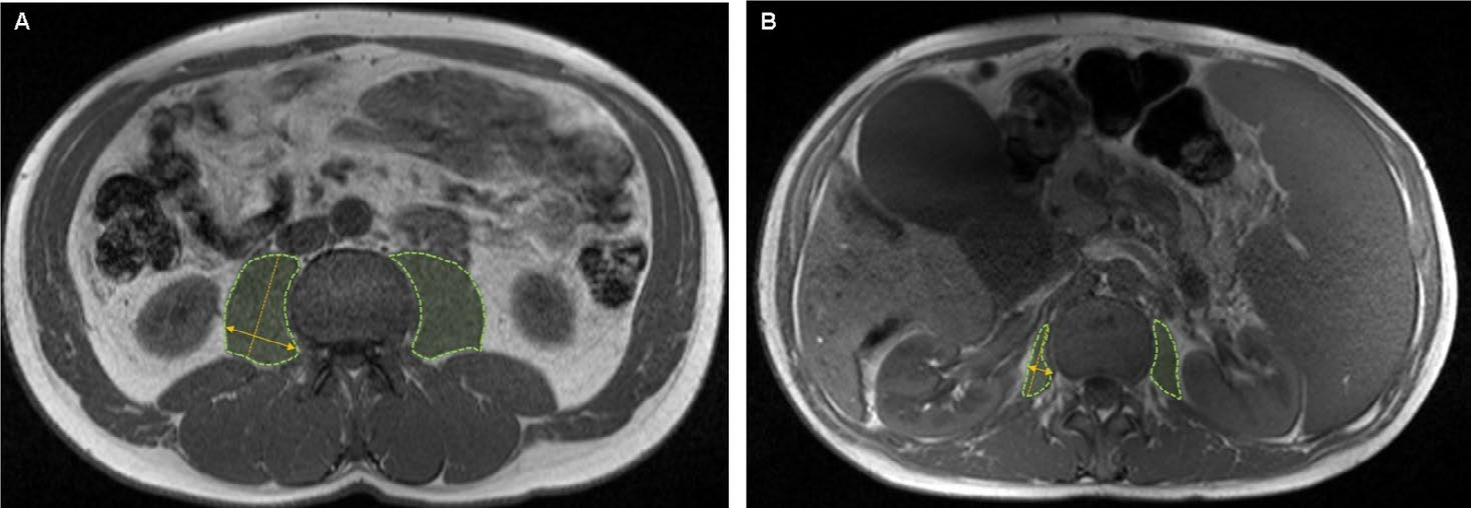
Muscle mass measurements were performed on a single slice at the level of the cranial endplate of the third lumbar vertebra. PMT: Psoas muscle thickness (yellow double arrow) was defined as the greatest diameter of the right psoas muscle perpendicular to the long axis (anterior–posterior oblique; yellow dashed line) and normalised to body height, as suggested by (20). PMA: Psoas muscle area (green area) was defined as the total muscle area of the right and left psoas muscle, as described previously by (15). (A) 37‐year‐old male PSC patient with PMT of 21.6 mm/m, PMA of 880 mm^2^ and uneventful follow‐up (7682 days). (B) 36‐year‐old male PSC patient with PMT of 4.9 mm/m and PMA of 563 mm^2^. 777 days after the MRI, the patient underwent liver transplantation.
